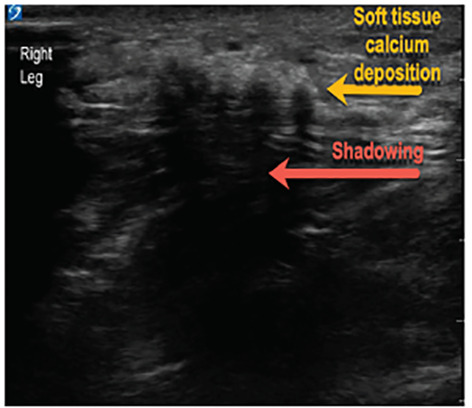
Soft tissue point-of-care ultrasound identifying soft tissue calcium deposits with shadowing diagnostic of calciphylaxis in a patient with end-stage renal disease.
